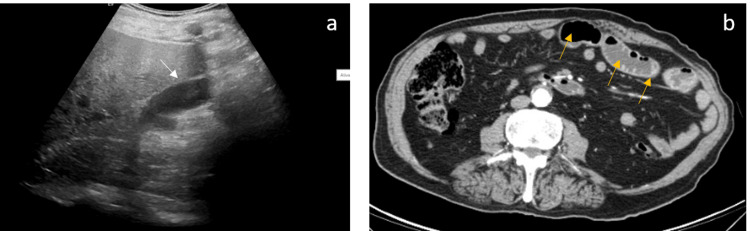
a) Abdominal ultrasound showing the gallbladder without lithiasis (white arrow) or fistulous tract; b) CT scan of the abdomen showing dilatation of the first jejunal loops with liquid and gas components and parietal thickening (yellow arrows)
